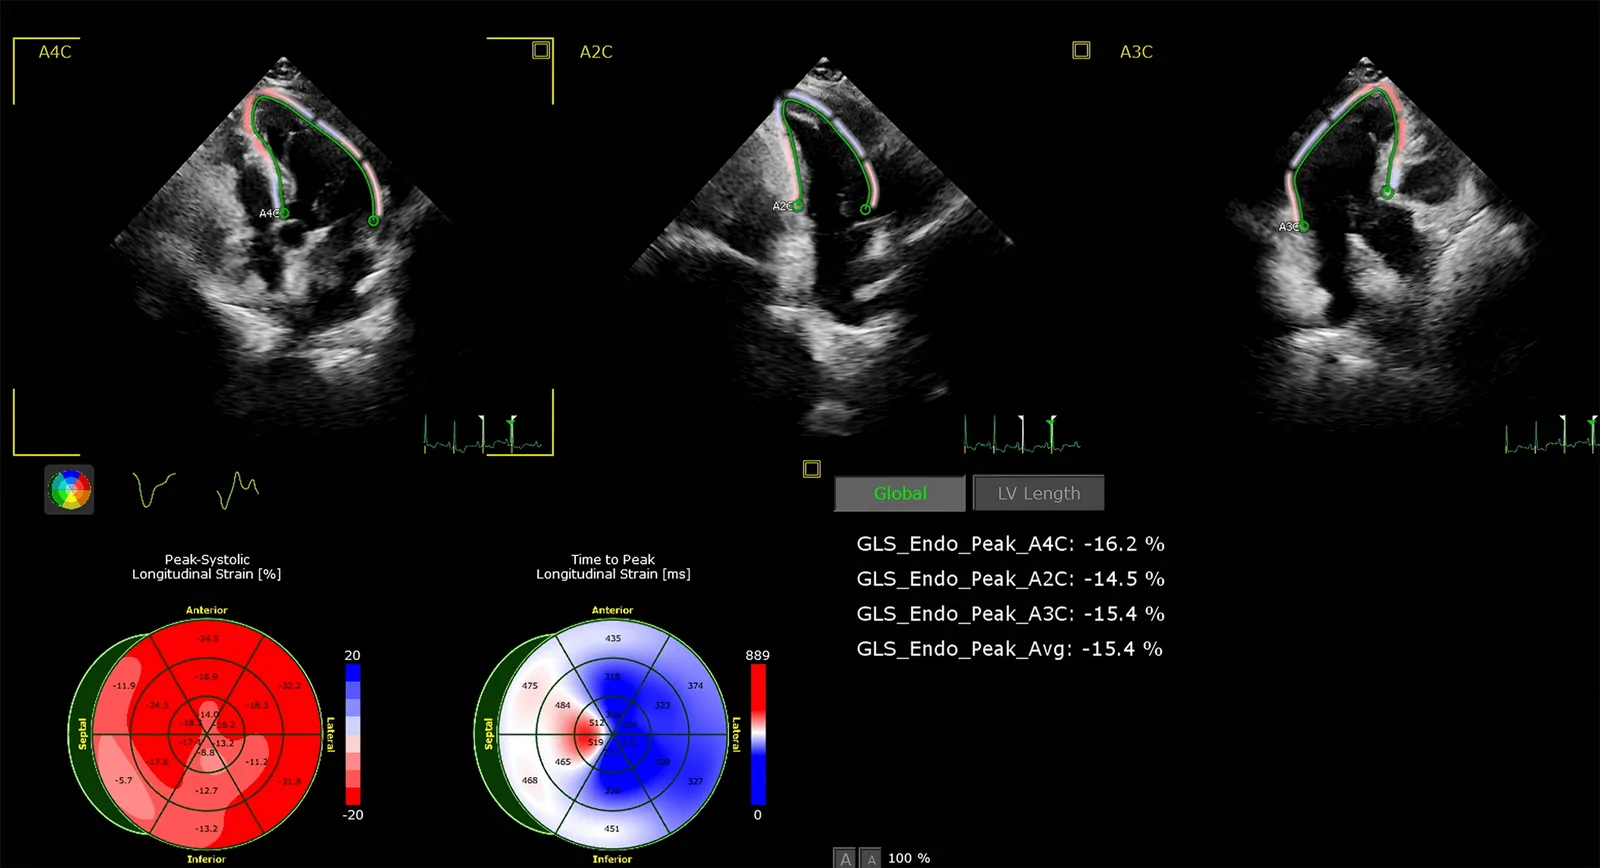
Eight-week follow-up two-dimensional speckle-tracking echocardiography (2D-STE) showing complete recovery of left ventricular longitudinal strain and normal cardiac function
